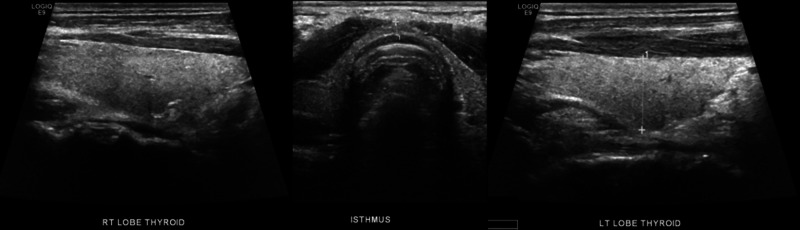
Ultrasound images showing no parathyroid lesions and a small nodule in the left lobe of an otherwise unremarkable thyroid gland
